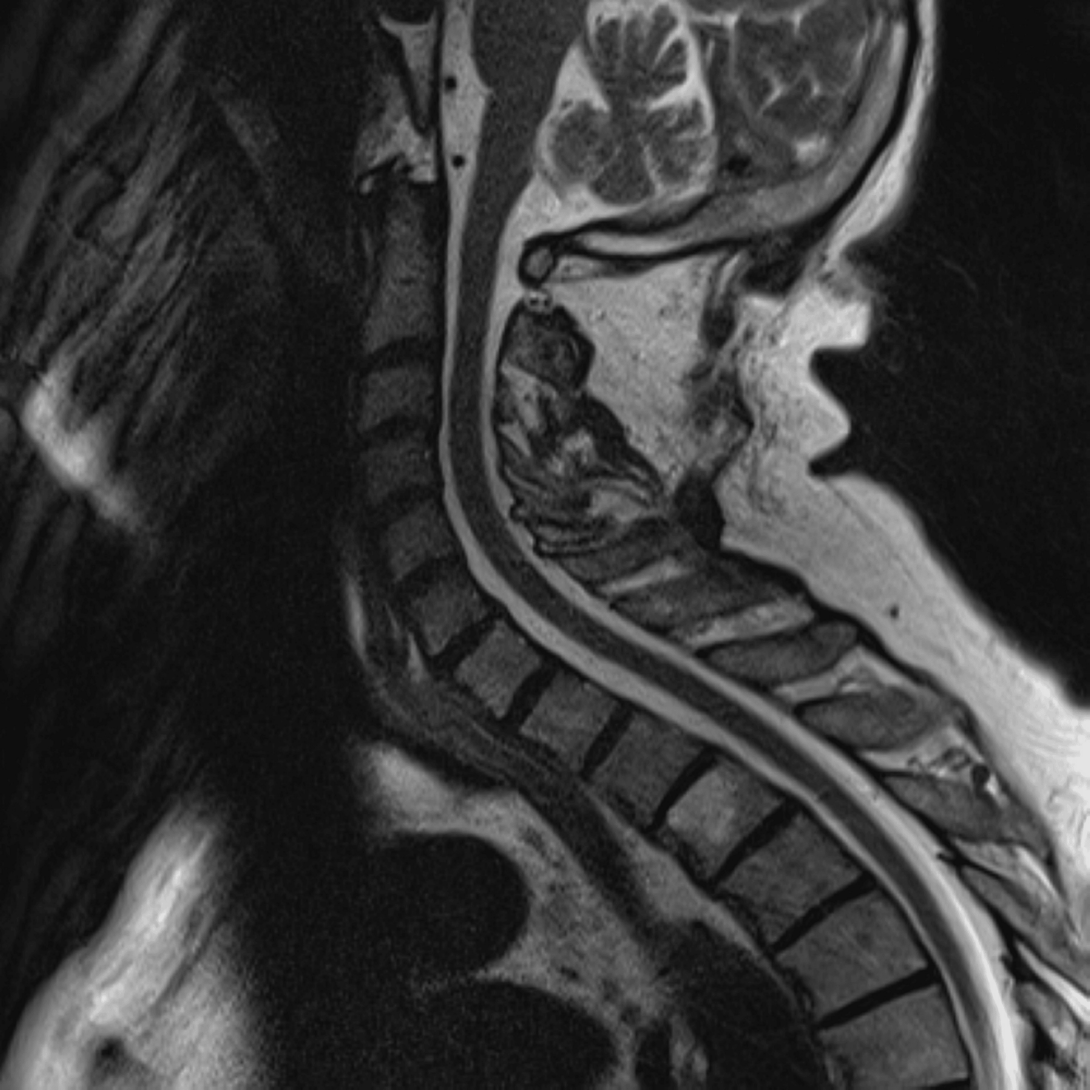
Cervical magnetic resonance imaging Absence of compressive discopathy, focal lesions or reduction in the vertebral canal that would justify the clinical setting.
